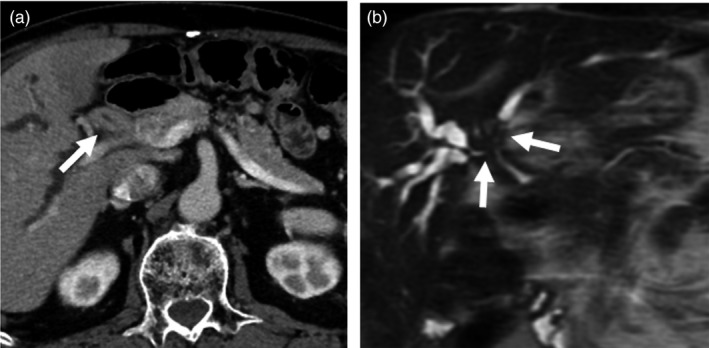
CT and MRCP images of IgG4‐SC. (a) In CT, the hilar bile duct shows marked thickening (arrow). (b) MRCP demonstrates bile duct strictures in the hilar bile duct (arrows)
